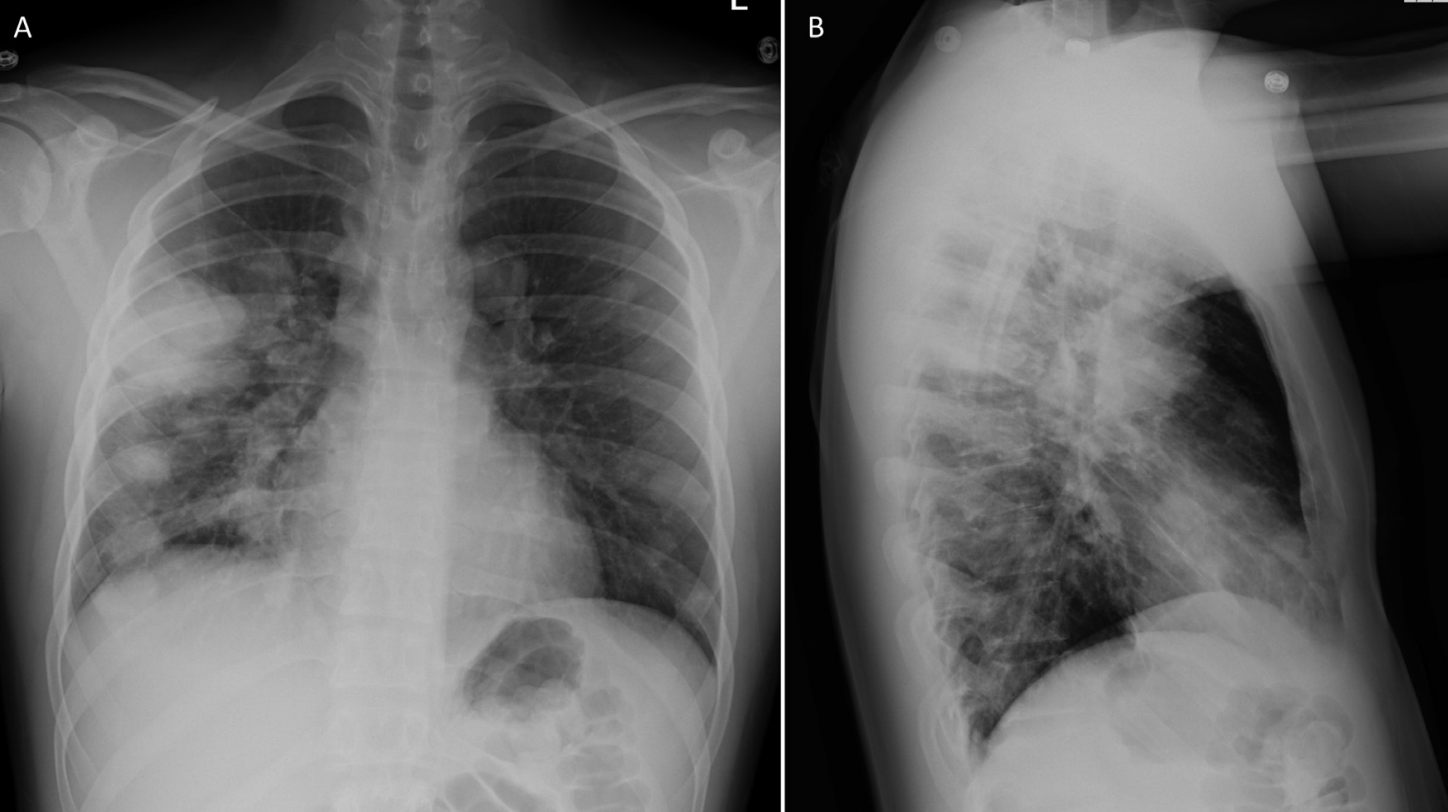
PA (A) and lateral (B) Chest radiograph demonstrates bilateral (right greater than left) multifocal nodular opacity, concerning for round pneumonia.
